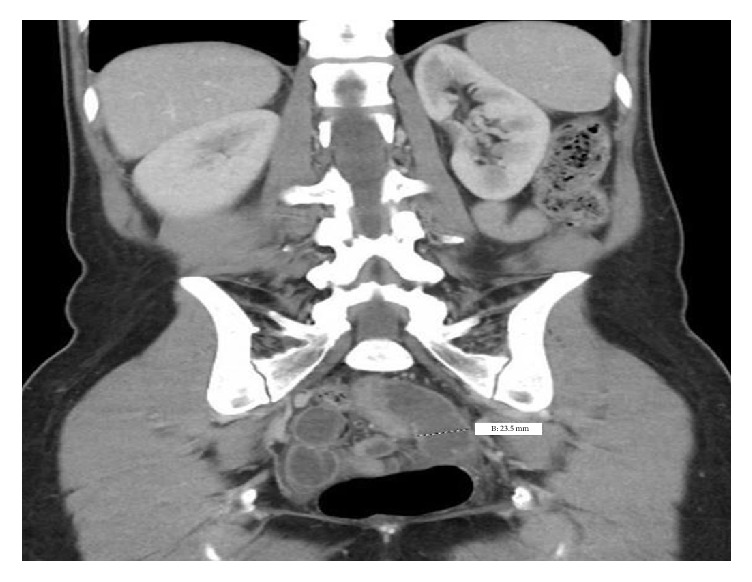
Coronal CT image of the small bowel extending into the vaginal. Normal thickness is less than 30 mm.
